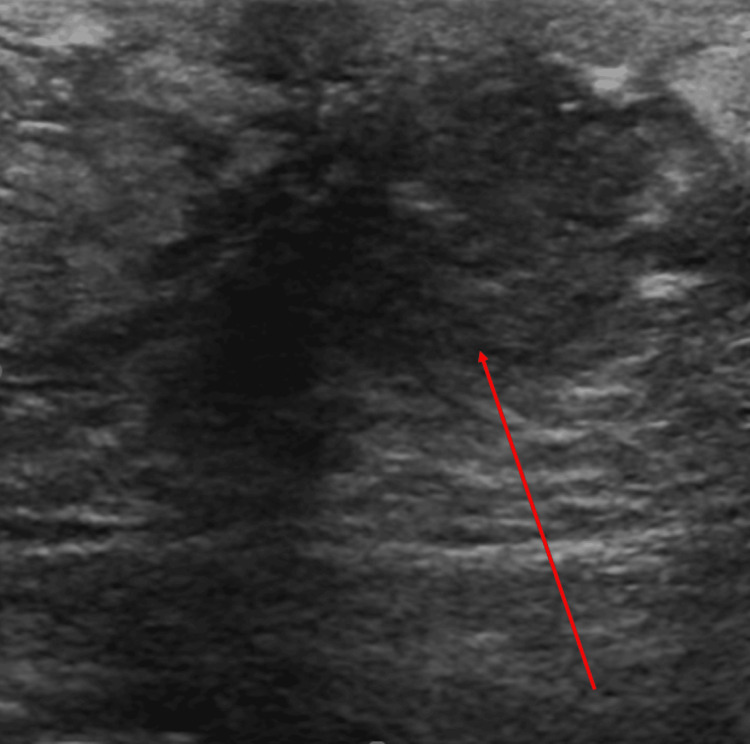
Targeted breast ultrasound showed a 6 × 4 millimeter smoothly marginated complex cystic lesion in the right breast at the 11 o'clock position (indicated by arrow).
